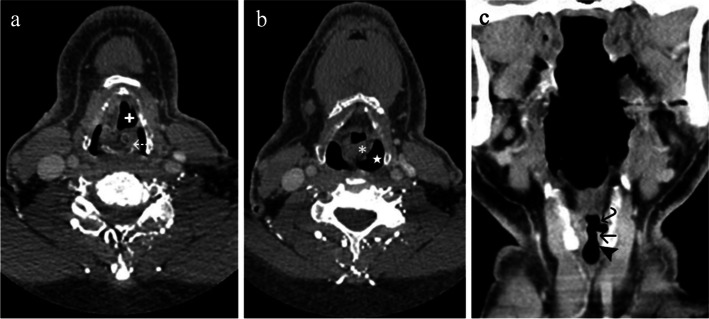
Contrast enhanced CT neck demonstrating indirect features of left RLN palsy. a Axial slice demonstrating ipsilateral laryngeal ventricle dilatation ( +) and medial rotation of the arytenoid cartilage ( ←). b Axial slice showing medialization of the aryepiglottic fold (*) and mild dilatation of the pyriform sinus (★). c Coronal reformat showing reduced bulk and pointed morphology of the vocal cord with loss of normal shouldering in the subglottic arch
